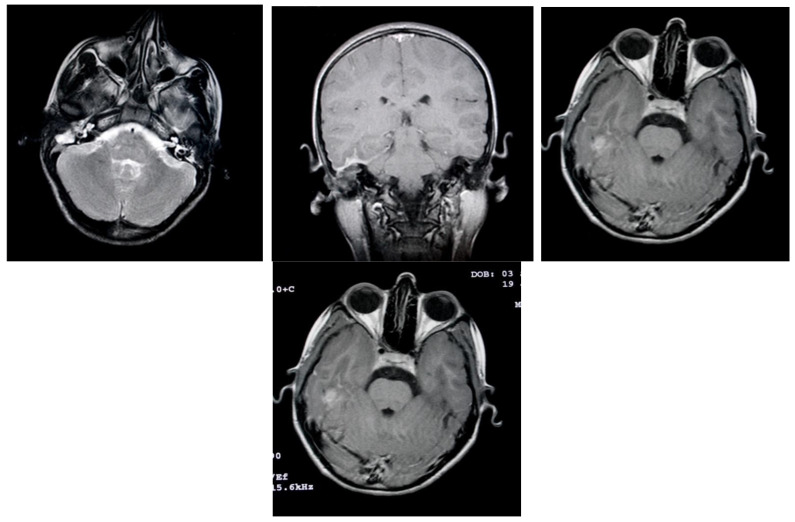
Patient with the diagnosis of acute cholesteatomatousotomastoiditis, right temporal cerebritis, and neighboring meningeal reaction in MRI examination with axial and coronal sections native and postgadolinium, an area in frank hyperseminal at the right mastoid, with fluid appearance; postgadolinium, diffusely demarcated area, at the level of the brain parenchyma, right temporal lobe, and meningeal pathologic contrast uptake, right temporal.
